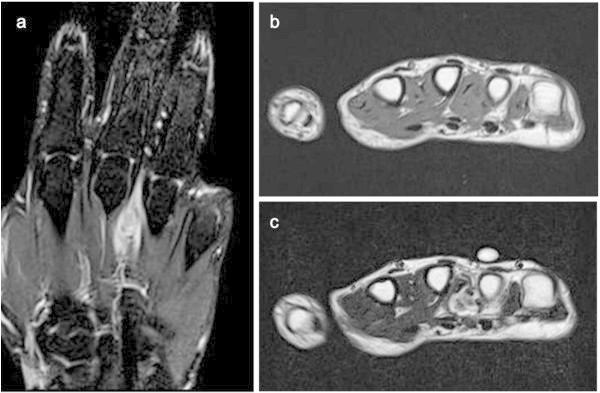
Recurrent synovial sarcoma in the dorsum of the hand: case 5. (a) MRI of the tumour showed high intensity on T1 fat sat image, (b) isointensity on a T1 image and (c) high intensity on a T2 image. The mass spanned the third and fourth metacarpal bones.
